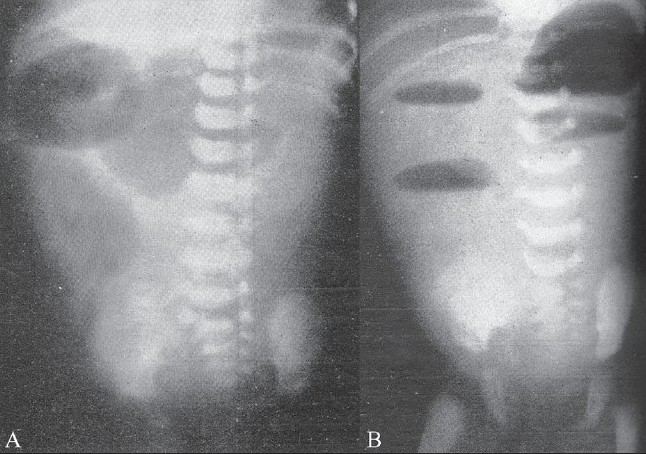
Supine (A) and erect (B) radiographs show a markedly dilated stomach and duodenal loop proximally in the supine position with air-fluid levels in the upright position
